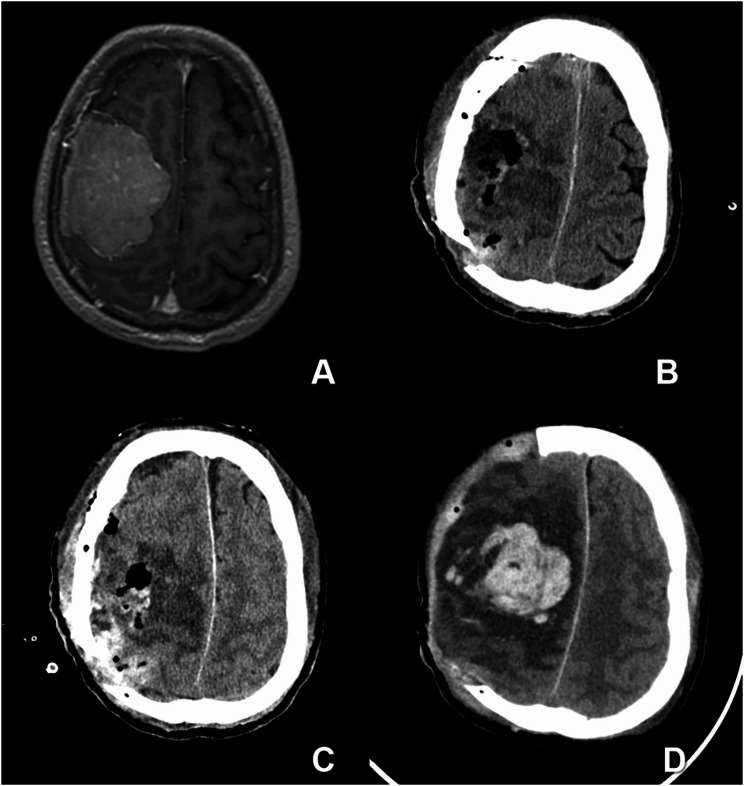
A 45-years old patient with postoperative progressive hemorrhage after right-frontal meningioma resection. (A) post-contrast T1WI before surgical rection, (B) CT examination immediately after surgical rection, (C) post-operation CT 6 h later, and (D) Follow-up CT two days later
